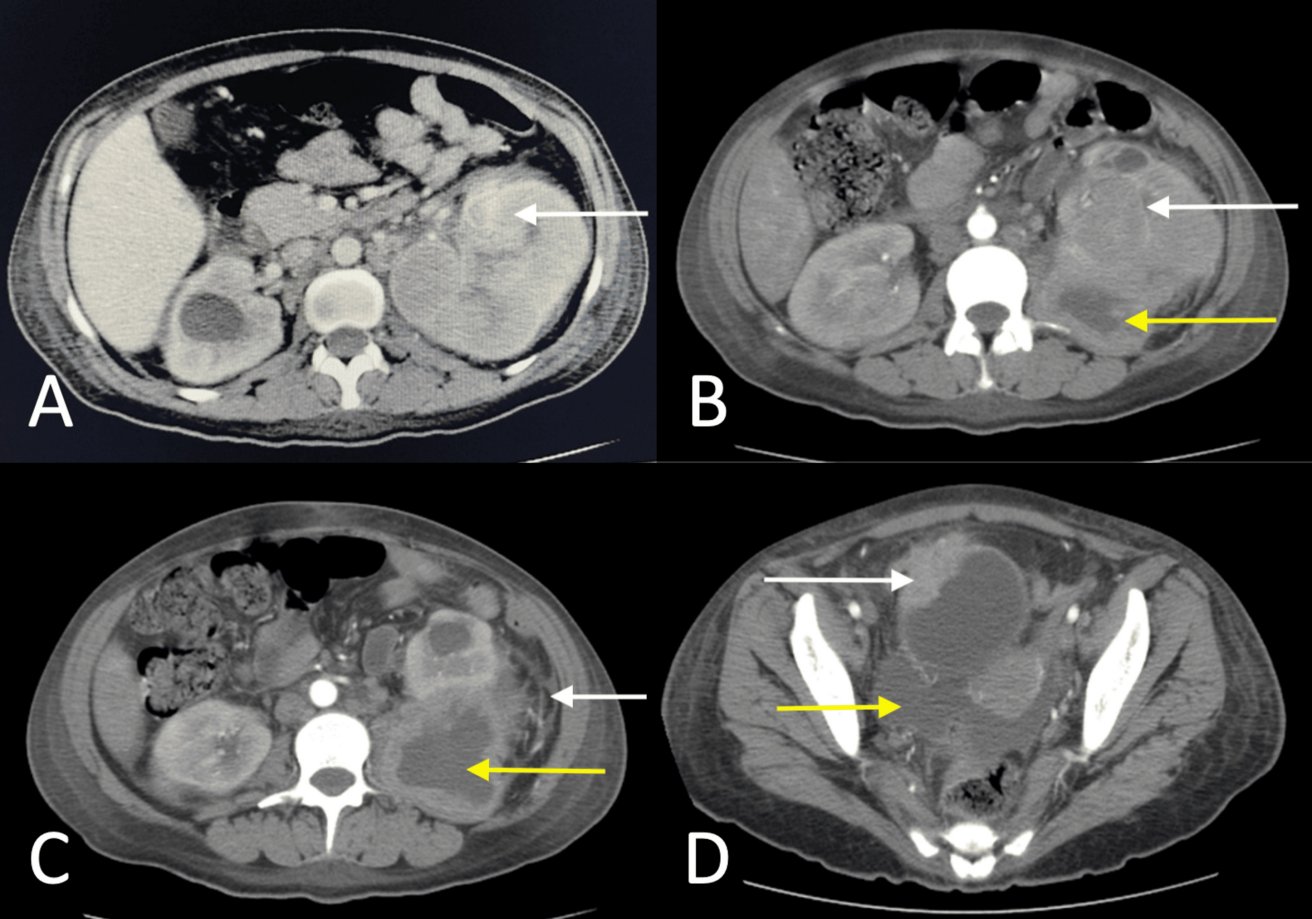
Contrast-enhanced axial CT images in the corticomedullary (A) and nephrographic phases (B, C, D). (A, B) Heterogeneous left renal mass primarily involving the mid and lower poles, with extension toward the upper pole (white arrows). The lesion shows heterogeneous enhancement, increasing from 15 to 50 Hounsfield Units (HU), with central non-enhancing areas suggestive of necrosis. The mass measures approximately 8.5 × 7 × 5 cm. Retroperitoneal lymphadenopathy is present. No definite evidence of renal vein invasion is seen. Abscess formation is noted in the lower pole (yellow arrow). (C) Perinephric fat stranding (white arrow) and abscess formation in the lower pole of the left kidney (yellow arrow). (D) Bladder tumor involving the right lateral wall with perivesical extension (white arrow), accompanied by ascites (yellow arrow).
